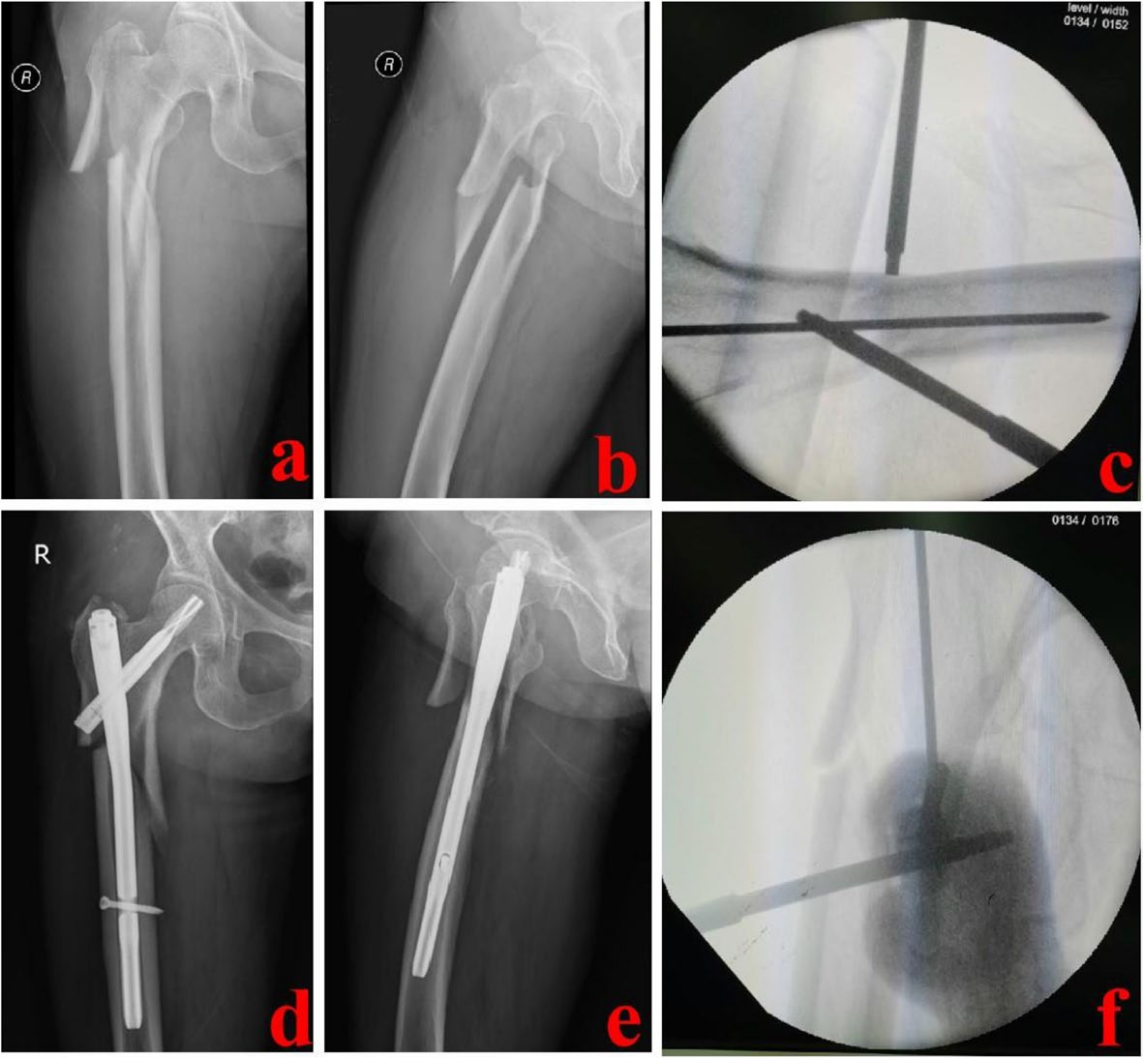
A 61-year-old patient was successfully treated with the PRDS technique. a and b: Preoperative X-ray images of the injured hip; c and f Intra-operative images of the PRDS technique; d and e Postoperative X-ray images of the affected hip. PRDS stands for percutaneous reduction with double screwdrivers
